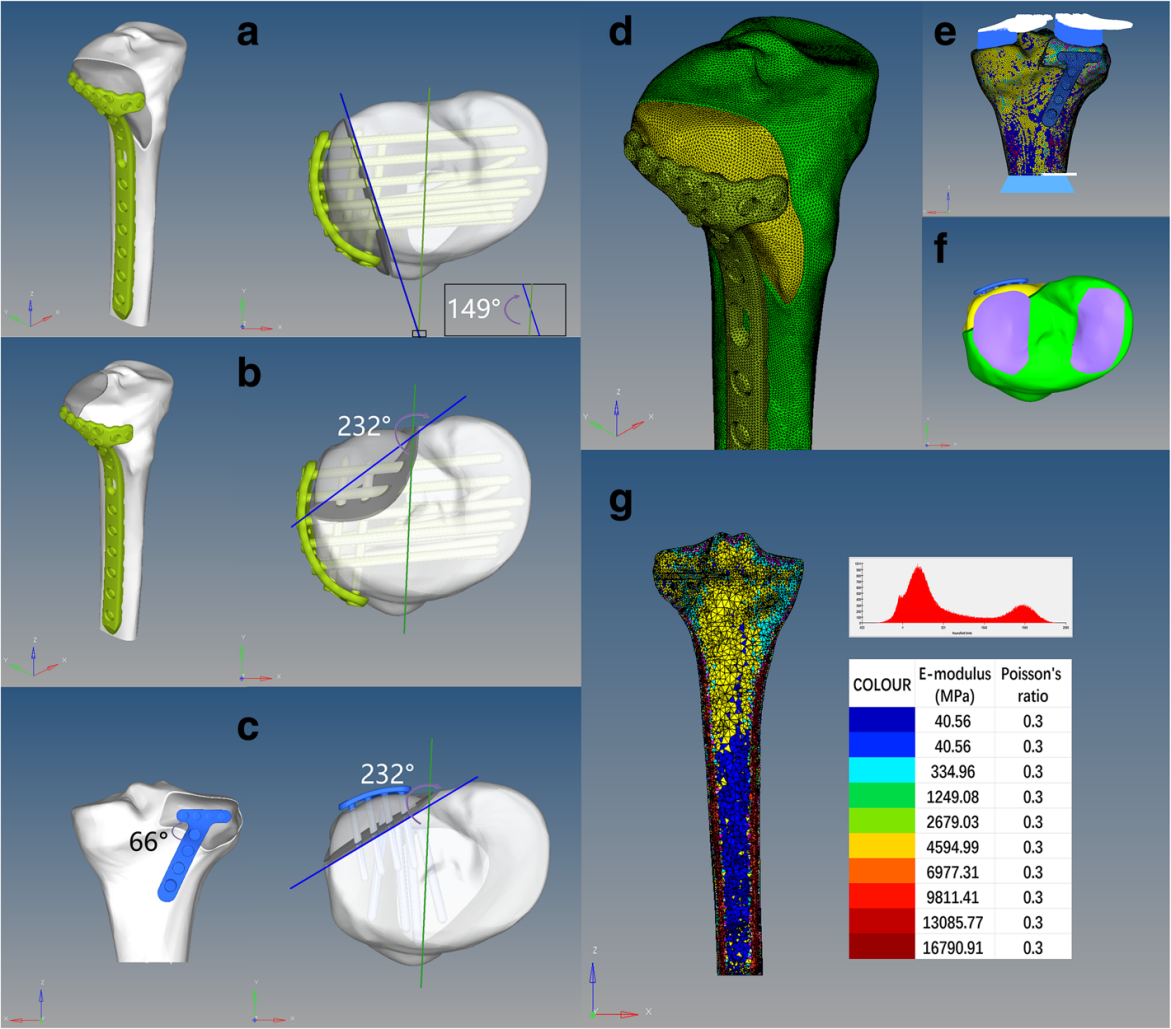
Brief introduction of the fracture models and the FE models. a SALF + ALP. b SPLF + ALP. c SPLF + PLP. d The FE model of SALF + ALP after meshing. e The FE model of SPLF + PLP with axial stress and constrain. f The location of axial stress. g A section of FE model after assigning the materials, the distribution of CT value, and the scale of materials. FLA: the green line connects the middle point of the posterior cruciate ligament’s insertion on the tibial plateau, and the medial 1/3 point of the tibial tuberosity was acted as a neutral axis; the blue line connects the two sides of fracture line
